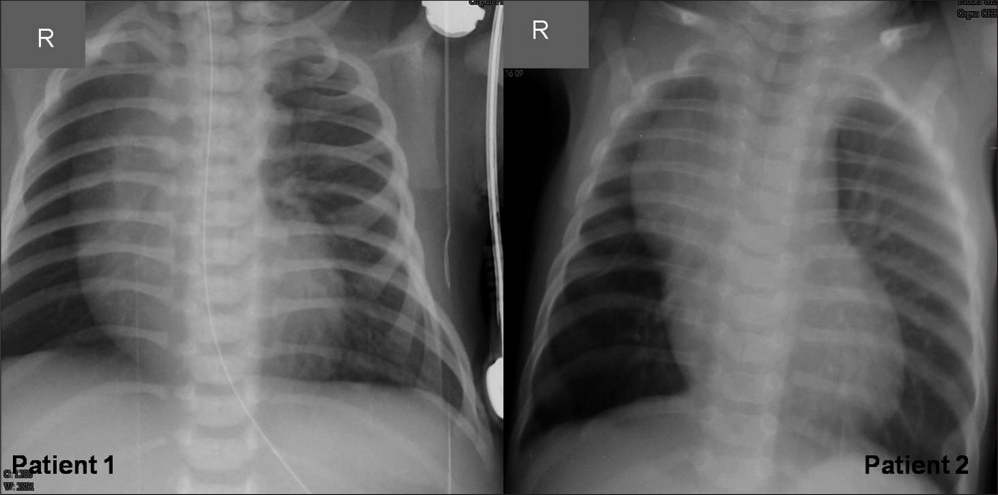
Chest X-ray (posteroanterior view) of patient# 1 and patient# 2 showing diminished hilar pulmonary artery shadow, a shrunken right lung and a shift of the mediastinal structures to the right side. Right-sided pulmonary vascular markings are diminished
